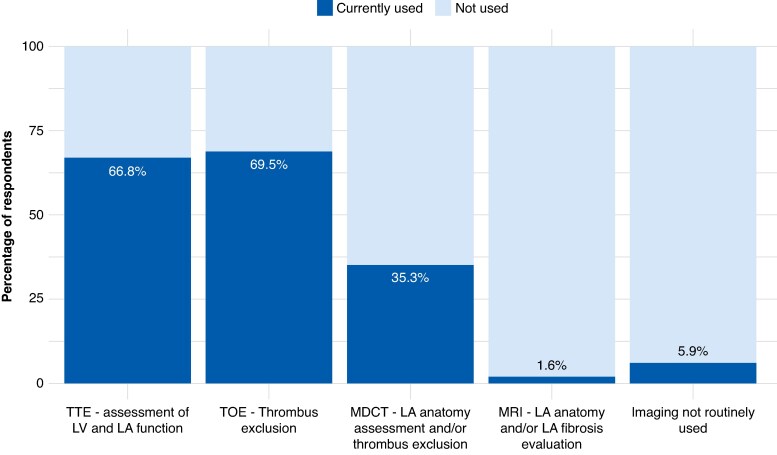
Pre-procedural cardiac imaging strategies in patients undergoing ablation for AAFl. Most respondents reported routine use of echocardiography (transoesophageal and transthoracic), whereas cardiac MDCT was used in roughly one-third of centres and late gadolinium-enhanced cardiac magnetic resonance was rarely employed. AAFI, atypical atrial flutter; LA, left atrium; LV, left ventricle; MDCT, multidetector computed tomography; MRI, magnetic resonance imaging; TOE, transoesophageal echocardiography; TTE, transthoracic echocardiography.
